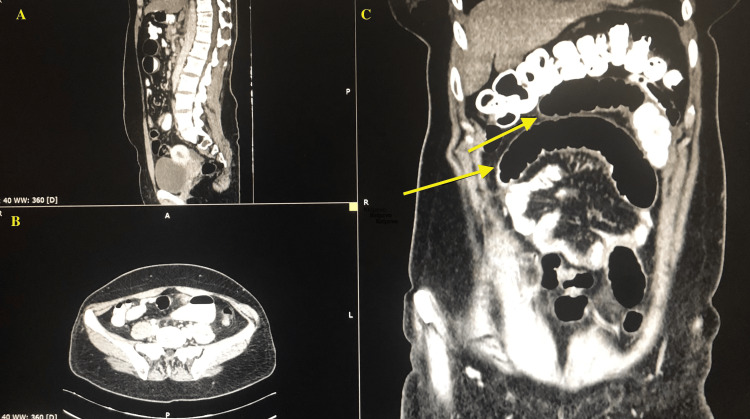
Abdominal CT scan revealing dilated small intestine loops (yellow arrows). A: Sagittal reconstruction. B: Axial reconstruction. C: Coronal reconstruction.
